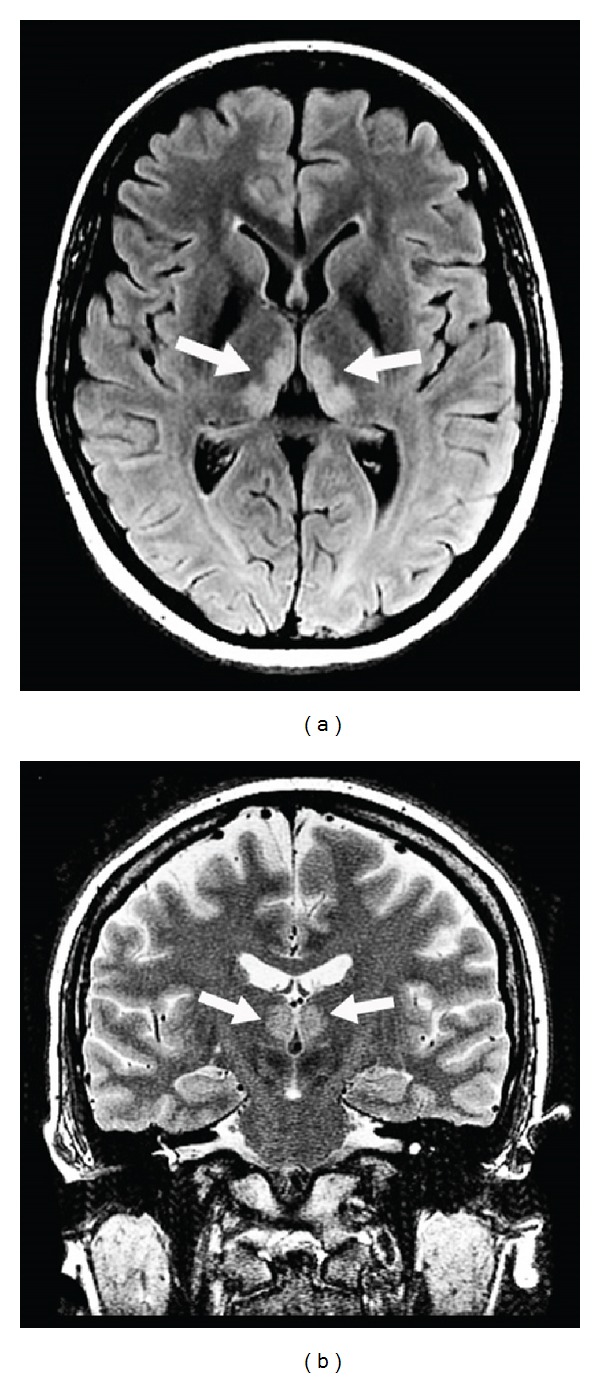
A patient with Wernicke syndrome showing hyperintensities in the medial part of the thalami on axial FLAIR (a) and coronal T2-weighted (b) imaging.
